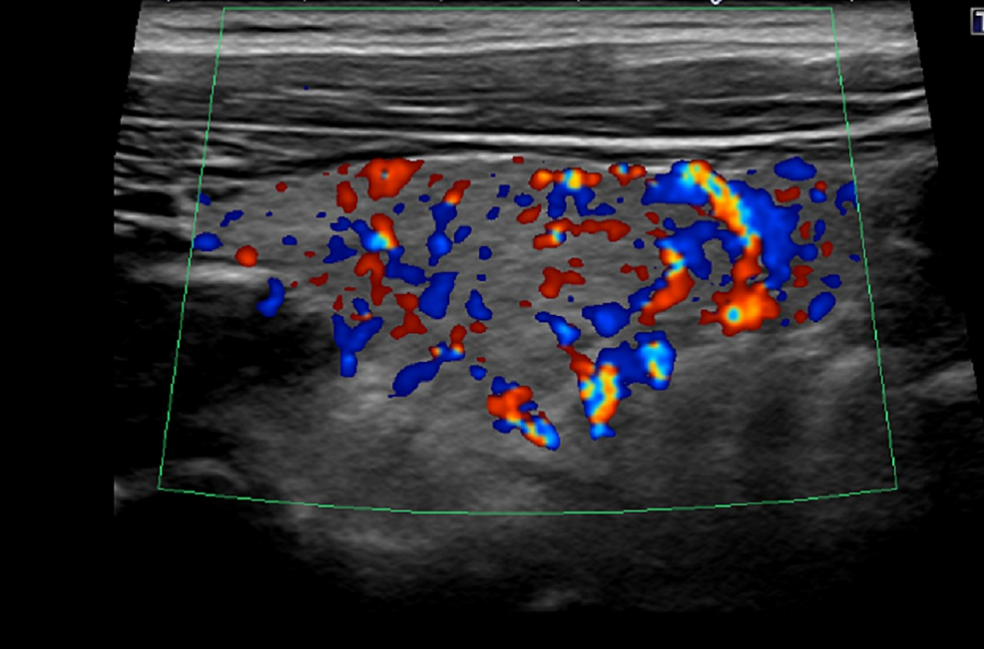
Thyroid ultrasound of Case 1: long axis with doppler showing increased vascularity in the entire right lobe
